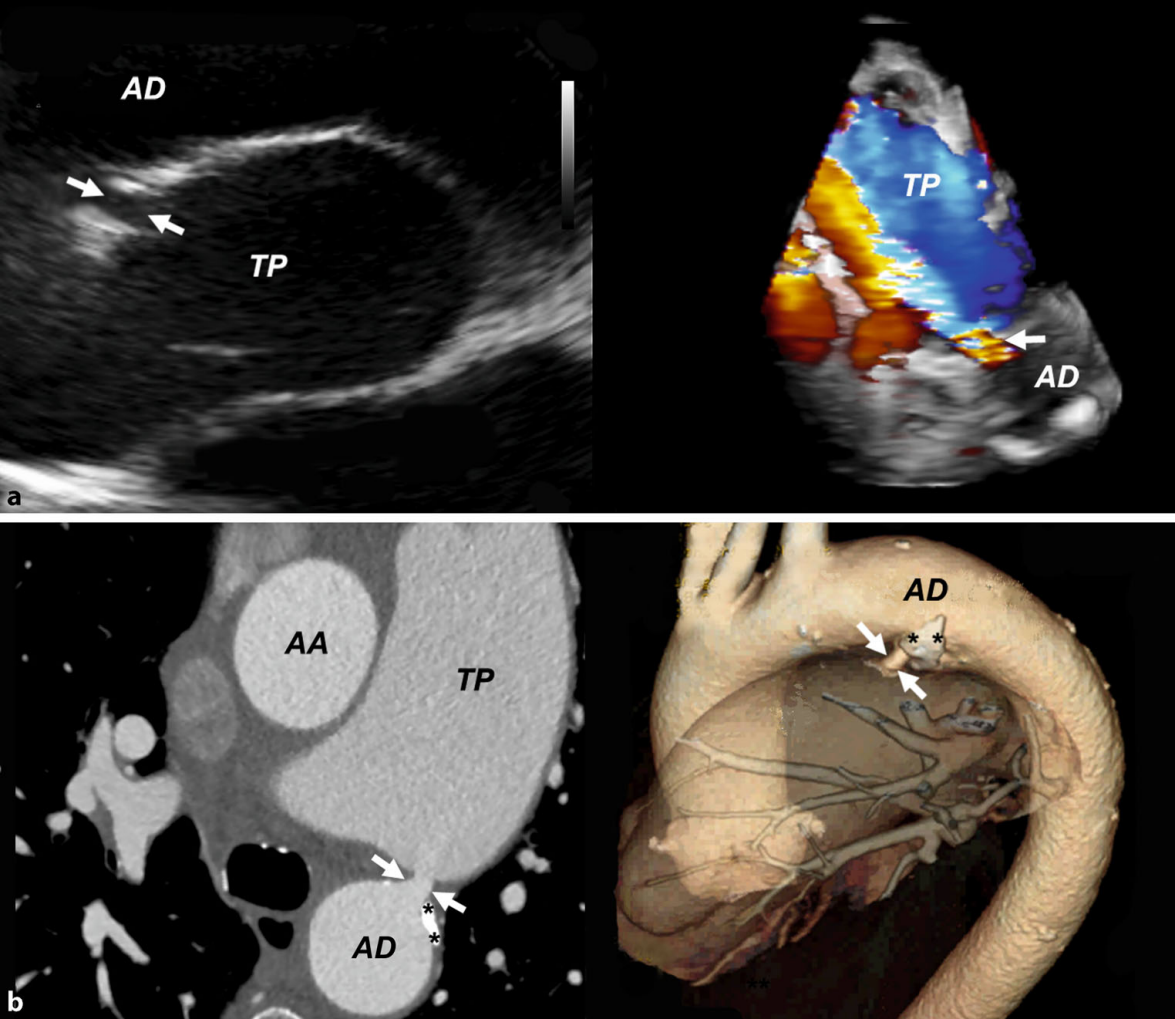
Multimodality imaging of patent partially calcified ductus arteriosus in an octogenarian female. *Upper panels* show images of transesophageal and 3‑D transthoraracic echocardiography, *lower panels* images of computed and 3D-VRT computed tomography. The diameter of the patent ductus arteriosus (*arrows*) was 6 mm, calcification is marked with asterisks (). *AA* ascending aorta, *AD* descending aorta, *TP* pulmonary trunk
